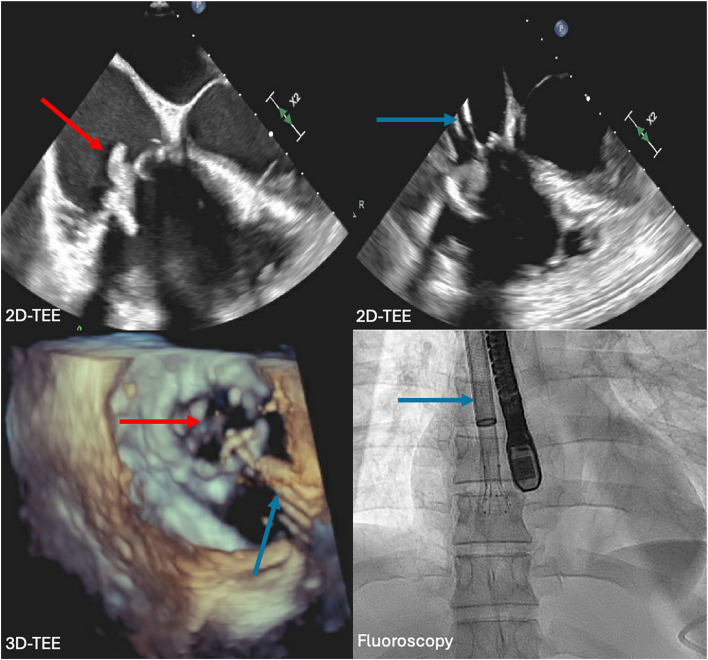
Transesophageal echocardiography (TEE), 2-dimensional (2D) and 3-dimensional (3D), and fluoroscopy images during percutaneous mechanical aspiration for tricuspid valve intervention. The red arrows indicate vegetation and the blue arrows indicate percutaneous suction cannula.
